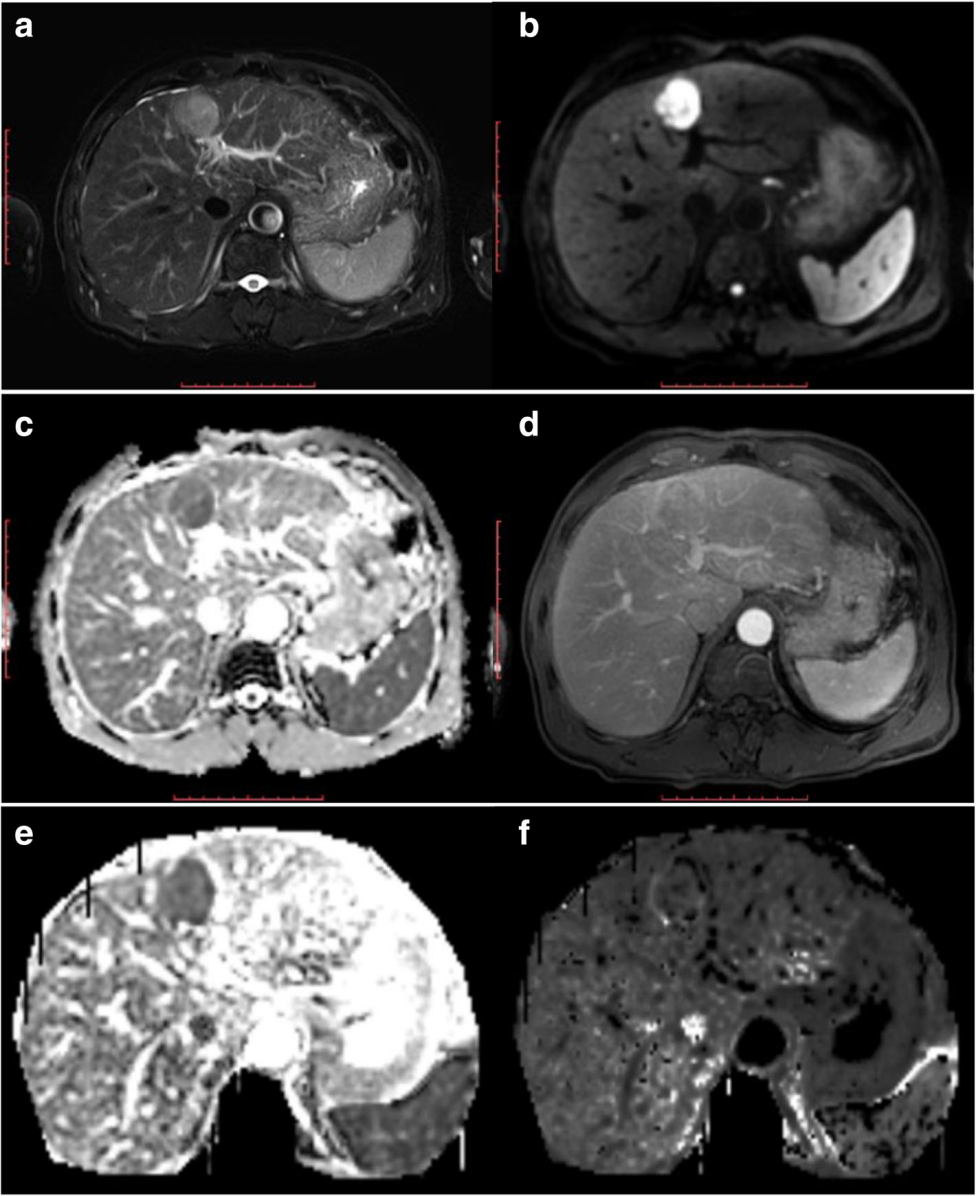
Images of a 48-year-old man with early stage single nodule of HCC. a axial fat-saturated T2WI image shows that the lesion of left hepatic lobe is slightly high signal intensity. b and c axial DWI and ADC map indicate that the diffusion of the lesion is obviously limited. d DCE arterial phase shows that the lesion has mild enhancement. e DKI-MD map shows that the MD value for the lesion is 1.181 × 10^− 3^. f DKI-MK map shows that the MK value for the lesion is 7.721 × 10^− 1^
